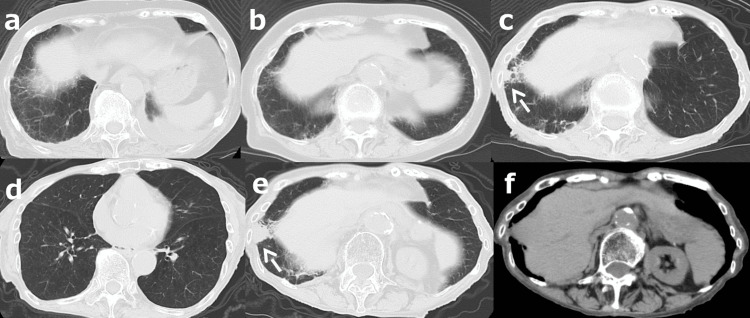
Clinical course as per CT A retrospective review of CT findings showed no mass in 10 (a) and 6 (b) years before the final admission and a tiny right pleural mass shadow (arrow) 1.5 years before the final admission (c), but no masses in either lung fields or the liver 8 months before final admission (d-f). The size of a right pleural mass (arrow) has become larger (e) CT: computed tomography
